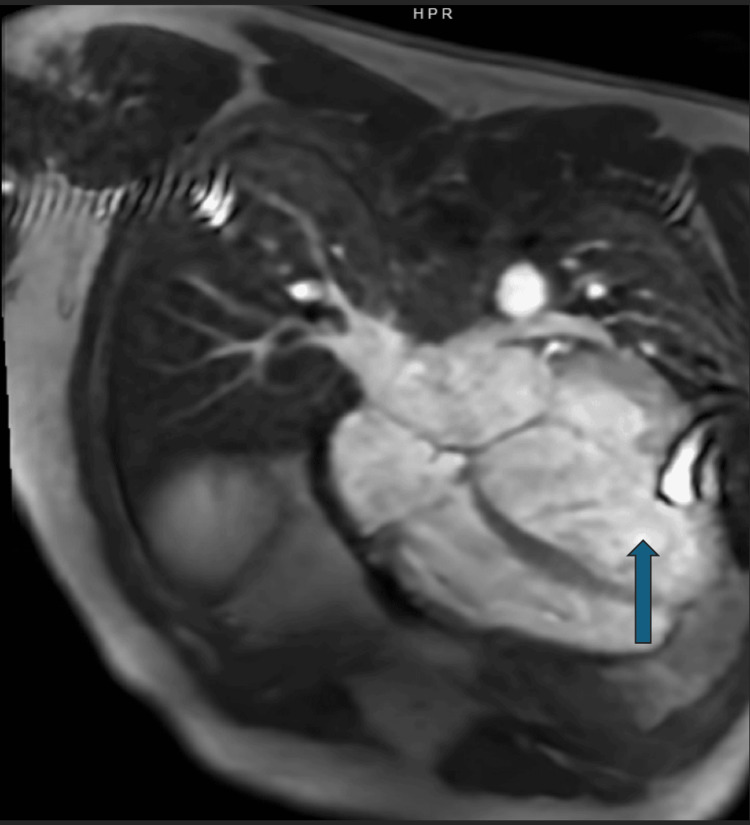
Cardiac MRI demonstrating biventricular failure, with an LVEF of 6% and an RVEF of 17%. The blue arrow denotes a severely dilated left ventricle without regional wall motion abnormalities. LVEF, left ventricular ejection fraction; RVEF, right ventricular ejection fraction
